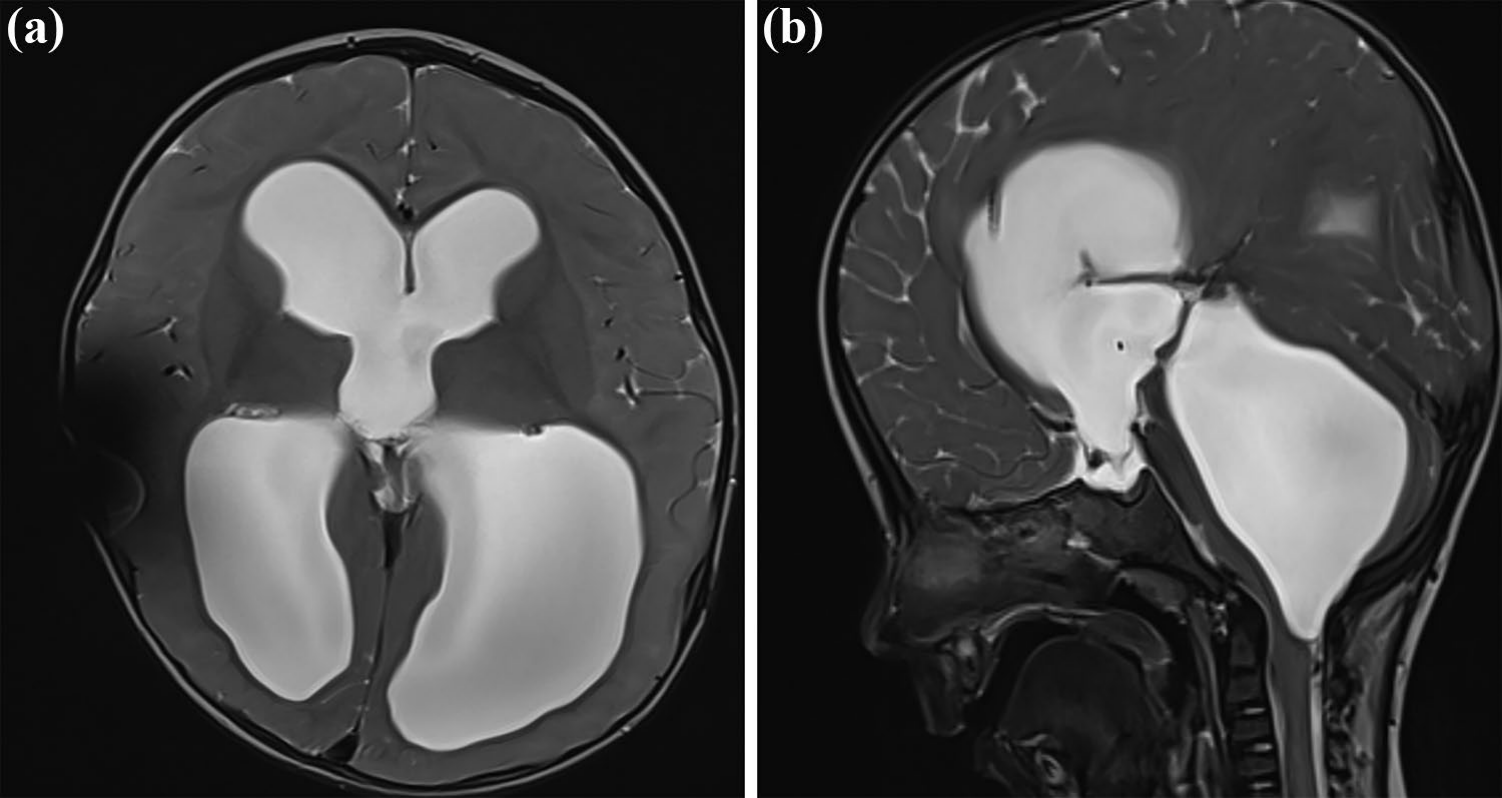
Preoperative MR images. Axial T2—(a) and sagittal T2-(b) weighted MR images. Chronic hydrocephalus with an aspect of isolating fourth ventricle after shunting
